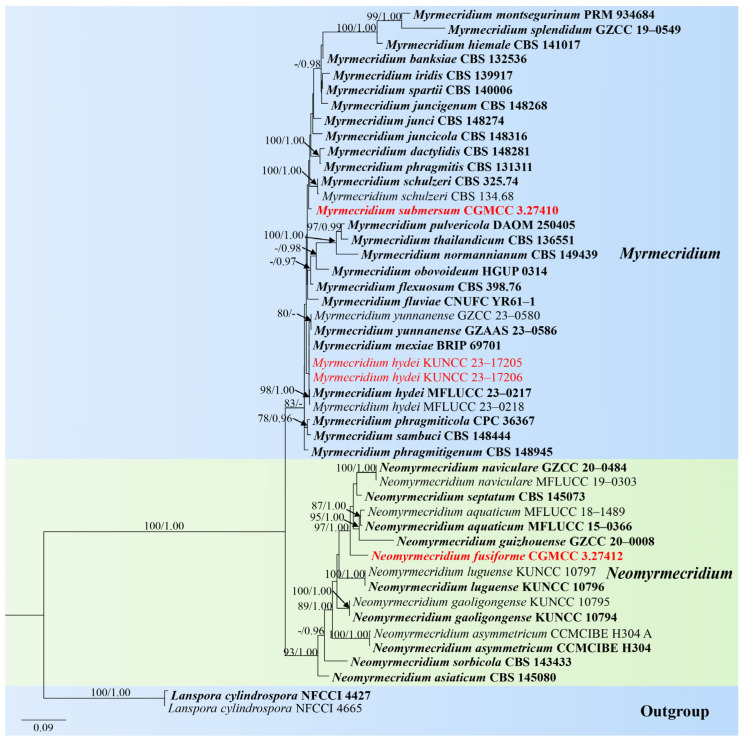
RAxML tree based on analysis of combined ITS and LSU dataset. The combined analyses include 47 strains with 1440 characters, including gaps (ITS: 556 bp and LSU: 884 bp). The tree is rooted to *Lanspora cylindrospora* (NFCCI 4427 and NFCCI 4665). The tree topology of the maximum likelihood analysis and Bayesian analysis are similar. The RAxML analysis of the combined dataset yielded a best-scoring tree with a final ML likelihood value of −7880.769866. The matrix had 543 distinct alignment patterns, with 8.41% undetermined characters or gaps. Estimated base frequencies were as follows: A = 0.245987, C = 0.247875, G = 0.286440, and T = 0.219698; substitution rates AC = 2.276747, AG = 2.342637, AT = 1.966718, CG = 0.662417, CT = 8.940493, and GT = 1.000000; and gamma distribution shape parameter α = 0.191988. Bootstrap values for maximum likelihood (ML) of ≥75% and clade credibility values of ≥0.95 from Bayesian-inference analysis are labeled on the nodes. The ex-type strains are in bold. The newly obtained sequences are indicated in red.
